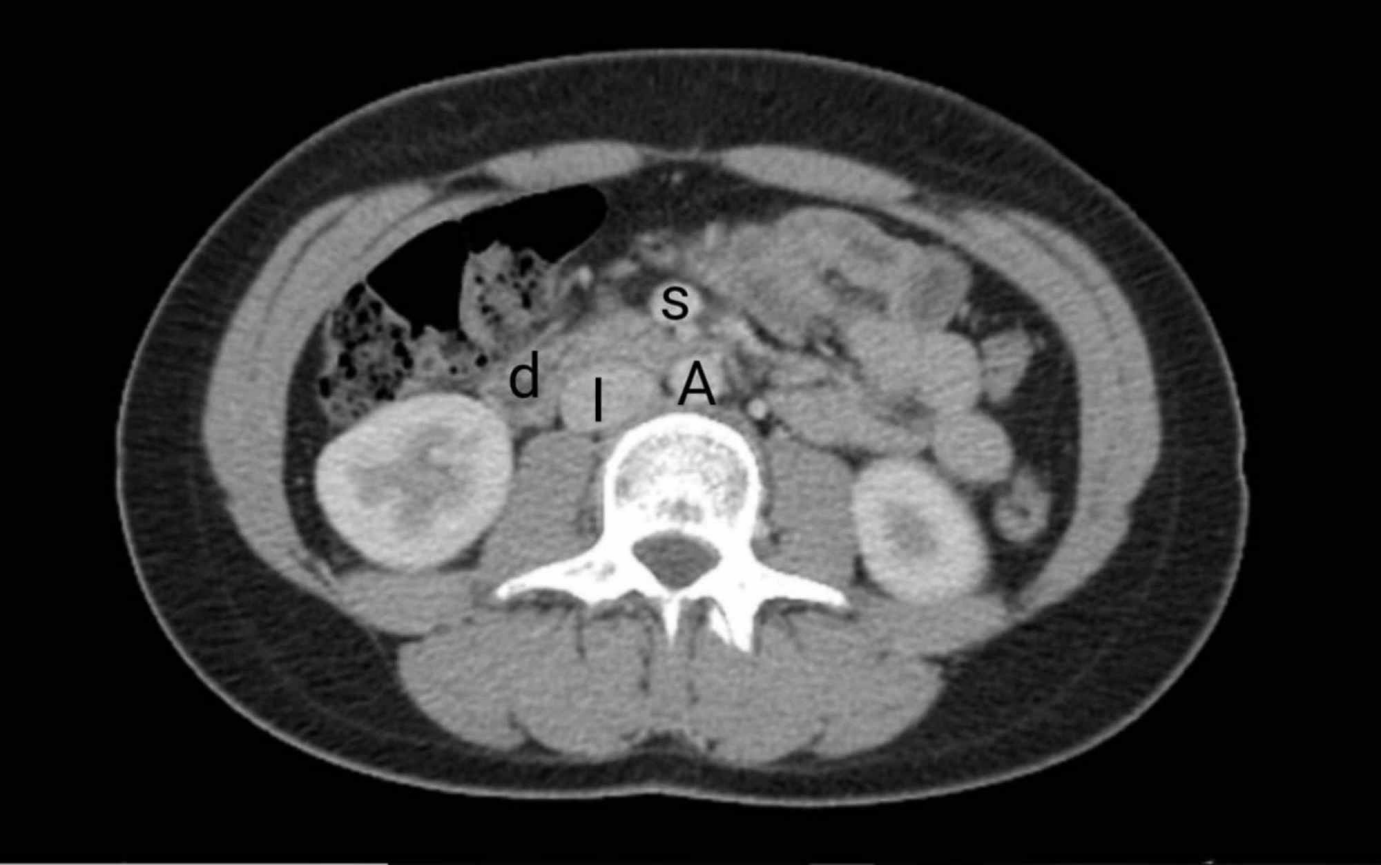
Computed tomography (CT) of the abdomen with contrast showing extrinsic compression of the duodenal segment (d) in between the aorta (A) and superior mesenteric artery (s) I: inferior vena cava.
